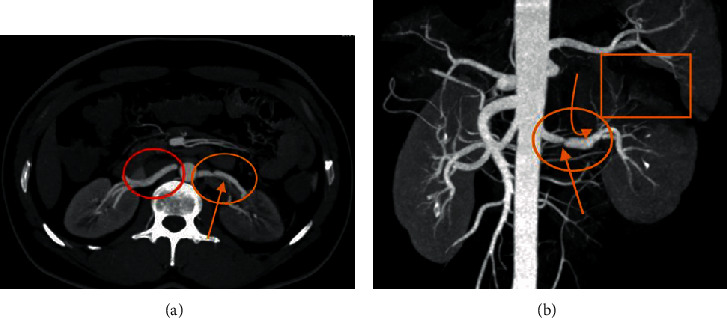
(a) Axial MIP CT image and (b) anterior volume-rendered 3D MPR coronal image were obtained after intravenous contrast material in arterial phase demonstrates focal thrombosis in the mid-left main renal artery (orange circle) and dissection flap (straight orange arrow in a), causing significant stenosis (orange straight arrow in b) and near-complete nonopacification of the upper segmental branch of the left renal artery for a length of approximately 3–4 mm with narrowed caliber and attenuated flow in the rest of the segmental and subsegmental branches (angled orange arrow in b), resulting in patchy cortical nonenhancement, predominantly involving the upper half and few discrete areas in the lower half of the left kidney, suggestive of infarct (orange rectangular in b), and also demonstrates normal right renal artery (red circle in a).
